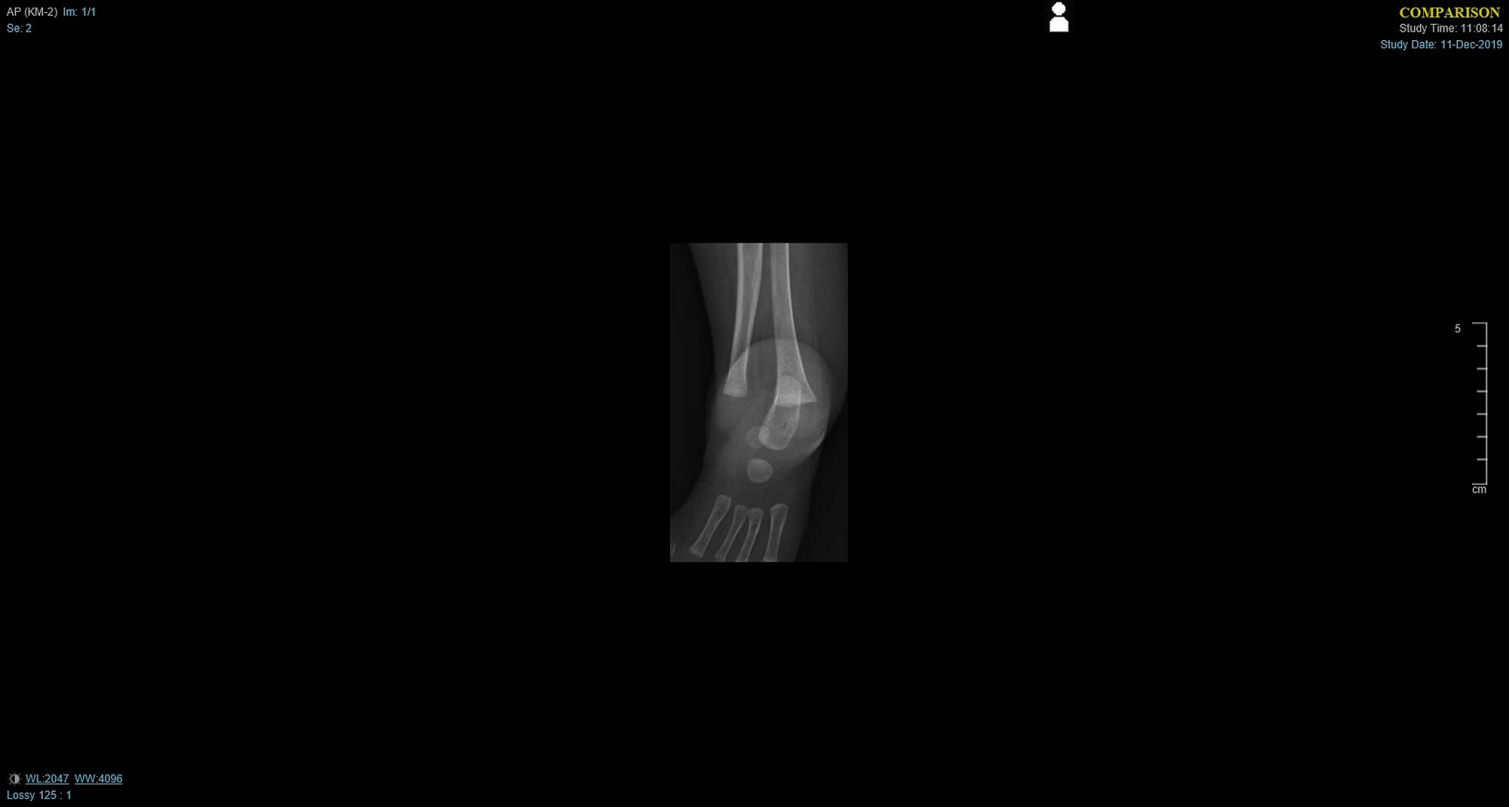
X-ray showing an underdeveloped left distal tibia and left distal fibula hypertrophy
